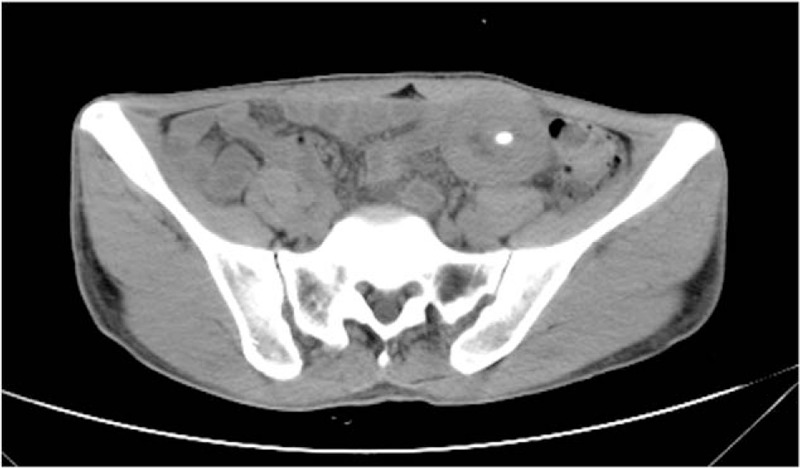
Transverse computed tomographic image showing the typical findings of postoperative gastrointestinal intussusception with feeding tube in the enteric cavity (high density shade).
